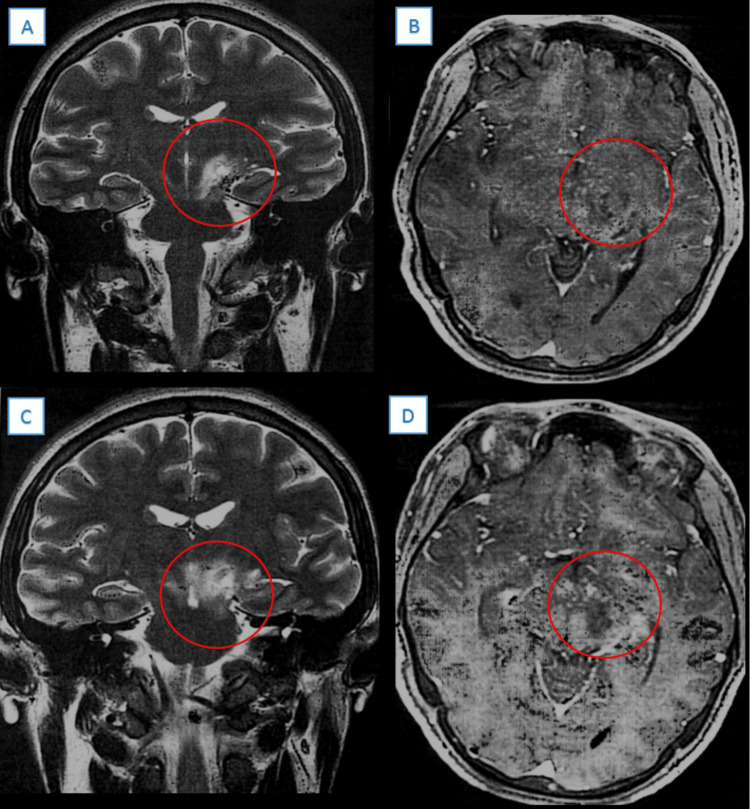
Cranial magnetic resonance imaging: (A) Coronal, T2; (B) Axial, T1; (C) Coronal, T2; (D) Axial, T1 A thalamic lesion (red circle), isointense on T1, and hyperintense on T2 with borders extending caudally toward the midbrain was observed.
